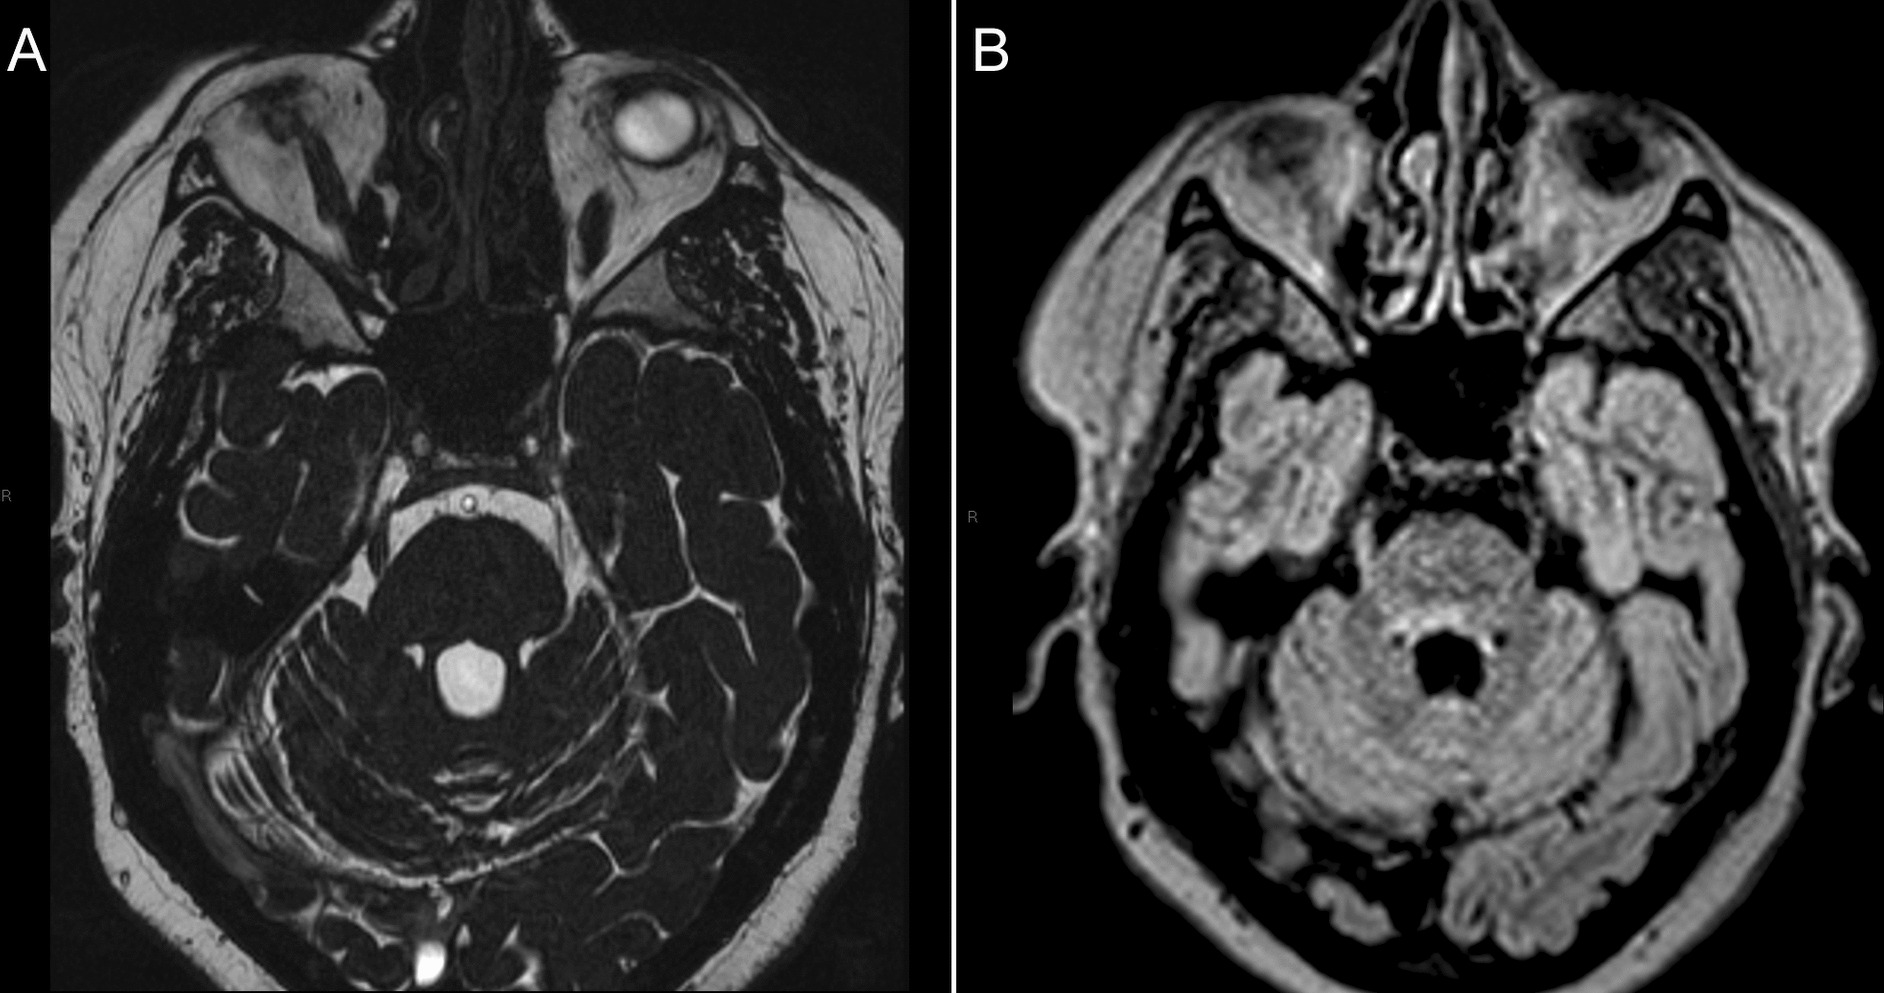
A Axial CISS sequence demonstrating the cisternal segment of the right trigeminal nerve without evidence of neurovascular compression. B Axial FLAIR image demonstrating hyperintense demyelinating signal within the brainstem extending toward the trigeminal root entry zone
